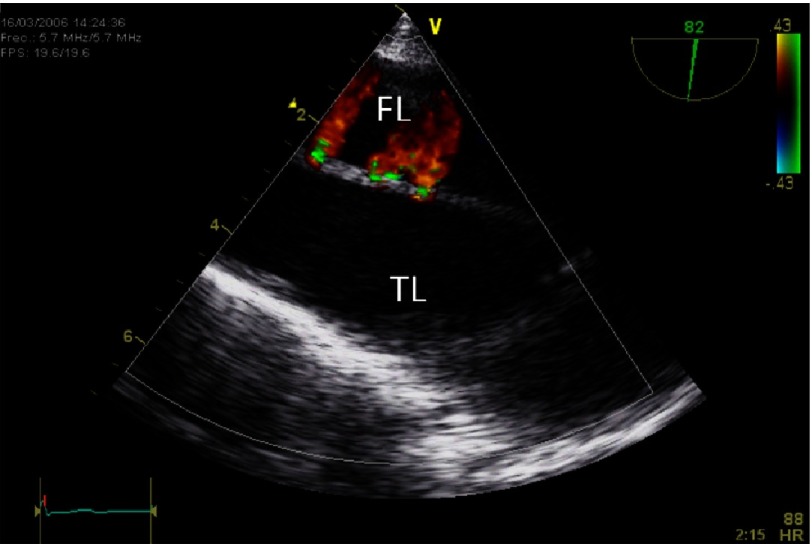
Transoesophageal echocardiography showing a type B AAD with secondary communications and small flows from true lumen (TL) to false lumen (FL).
